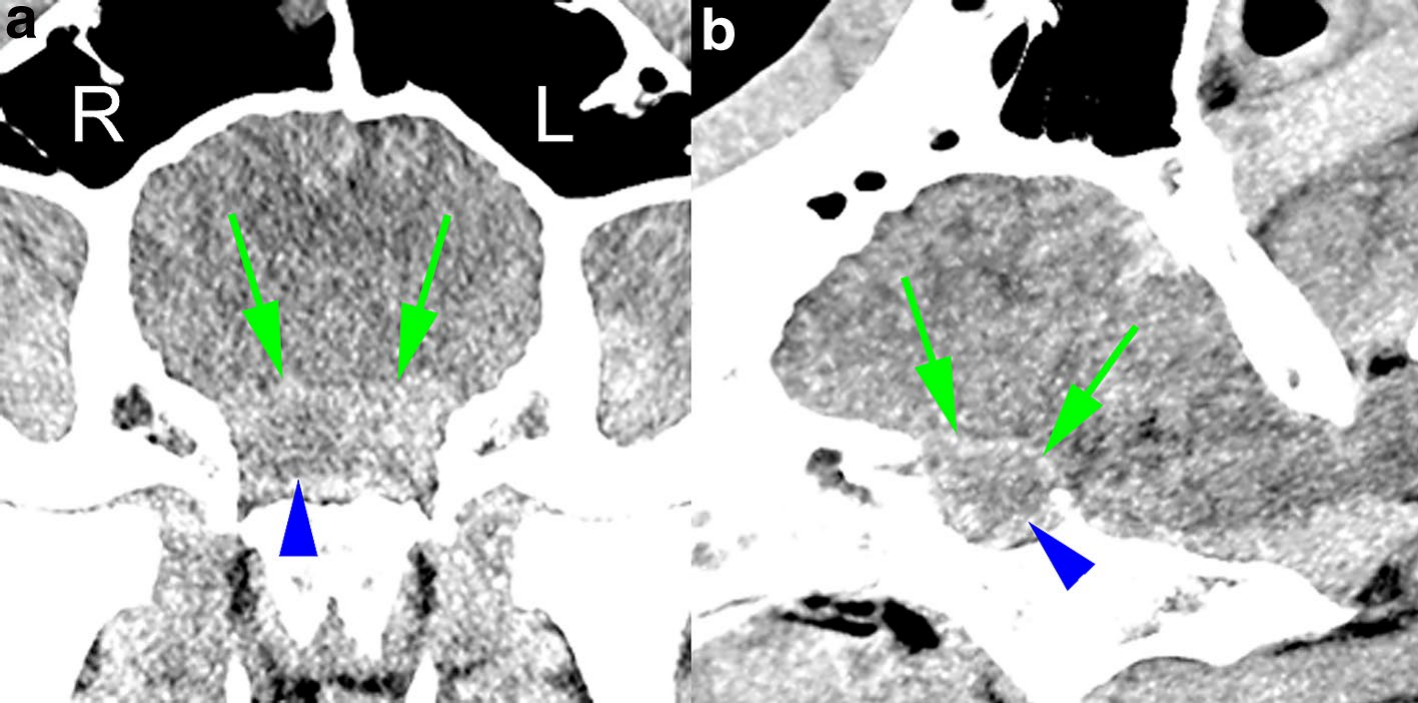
Contrast-enhanced CT image. Transverse a and sagittal b contrast-enhanced CT image reconstruction in a soft tissue algorithm (window level 50, window width 200) of the brain of a Holstein–Friesian bull with abscess of the hypophysis. The attenuation value of the hypophysis (*green arrows*) was higher than in the pre-contrast image. There is a cavity at the centre of the right side of the hypophysis with low attenuation (*blue arrow heads*). An abscess in the hypophyseal tissue is the most likely diagnosis of this cavitary lesion, whereas a Rathke’s cleft cyst or neoplasm is less likely
